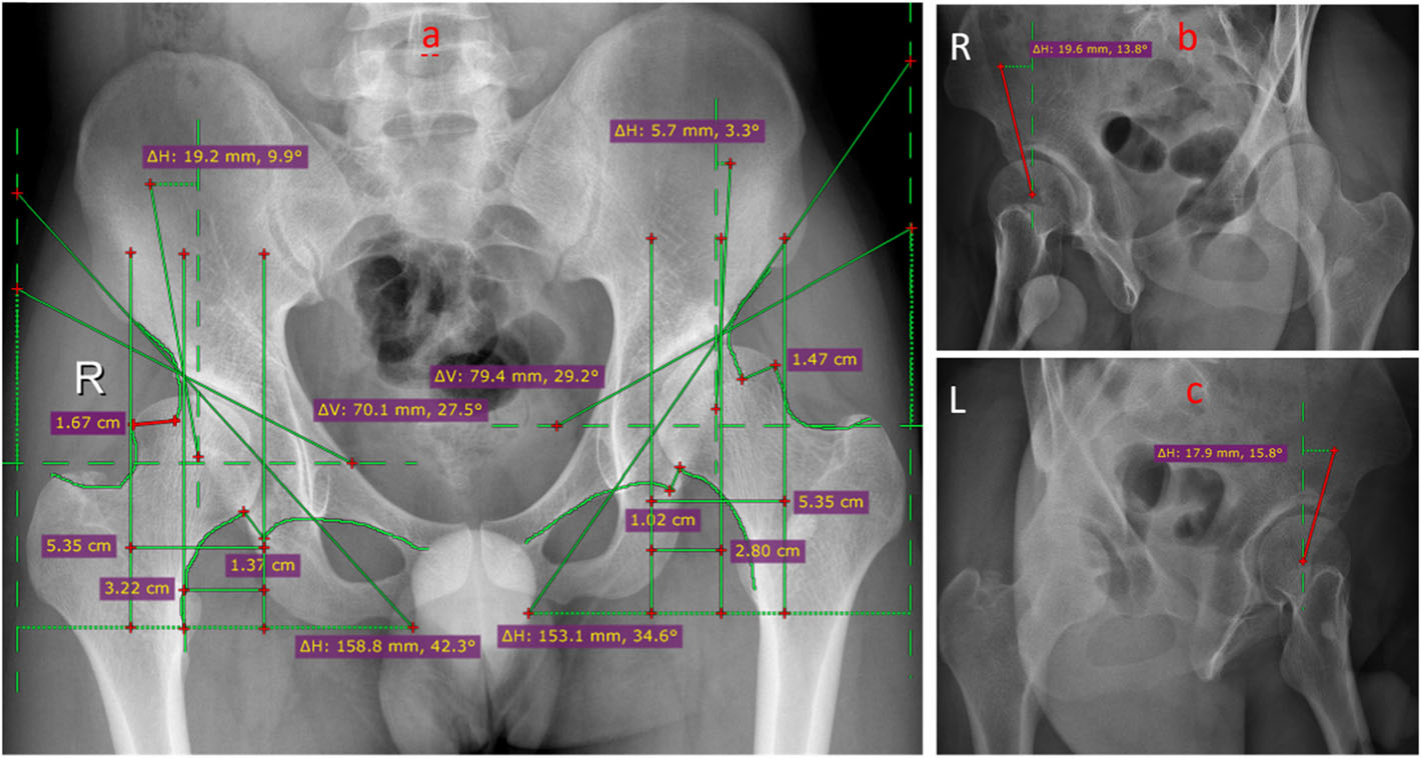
Radiographic images of the hip at pro-operation. a Pelvic positive position. b Left hip joint 65° oblique piece at pro-operation. c Right hip joint 65° oblique piece at pro-operation
